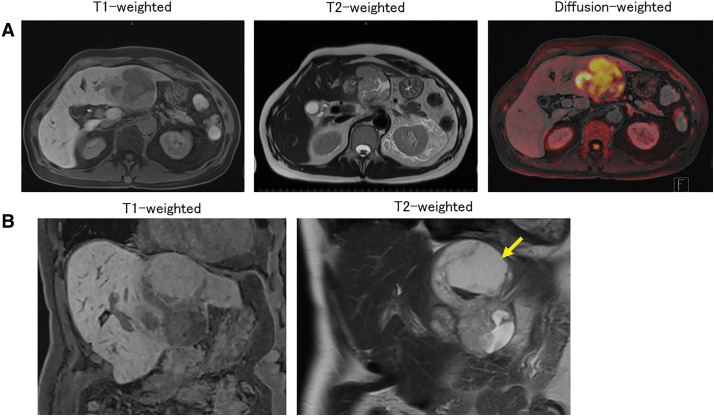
MRI findings for the tumor (A). Panel (A) shows axial magnetic resonance images of the tumor, which shows a low signal on the T1-weighted image, primarily a low signal along with a high signal area that appears to represent liquid on the T2-weighted image, and a high signal on the diffusion-weighted image (B). Panel (B) shows coronal magnetic resonance images of the tumor, showing a cystic and liquid component (yellow arrow) on the head side.
